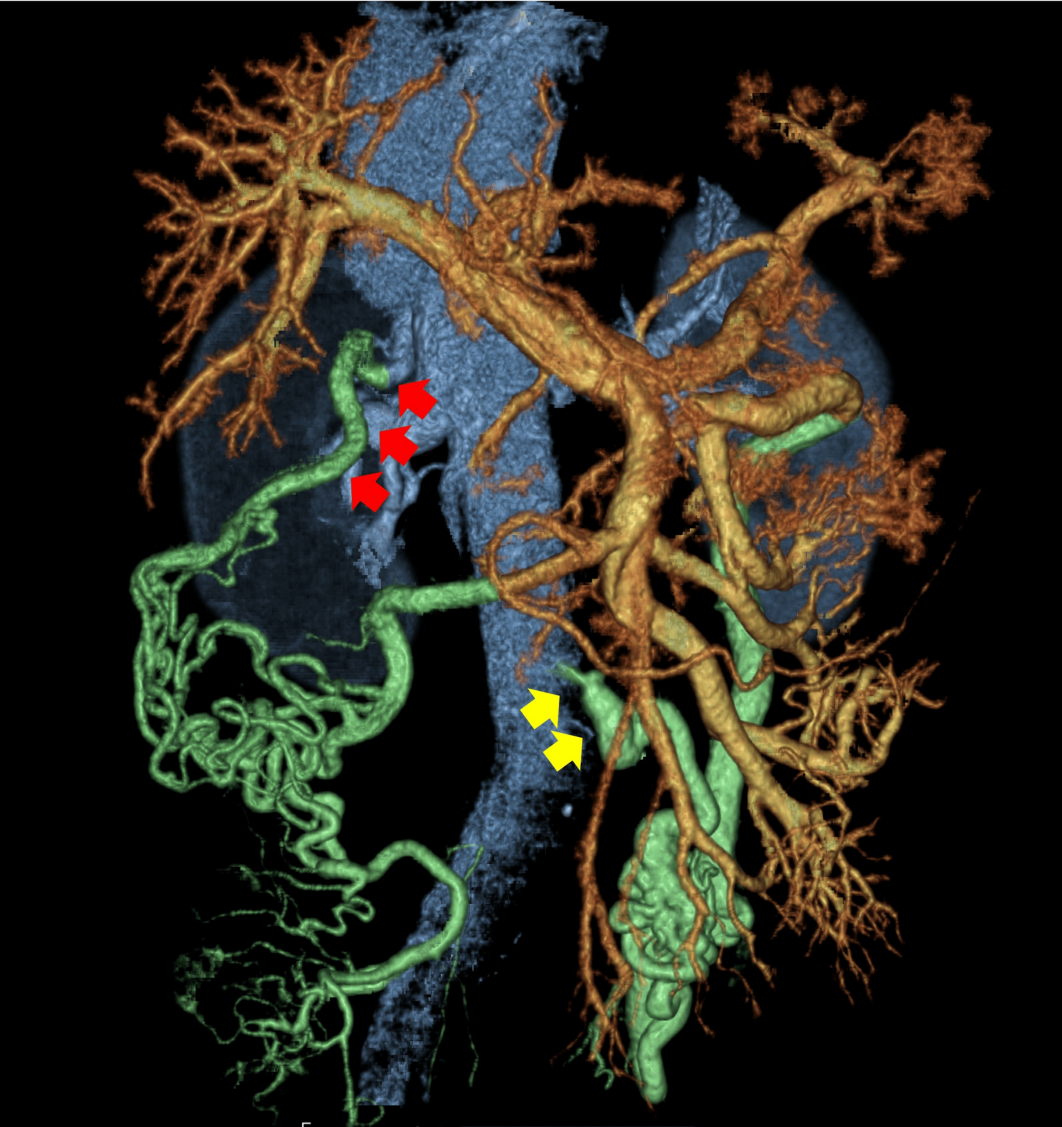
Contrast-enhanced CT Contrast CT showing abnormal blood vessels draining from the portal system into the inferior vena cava (yellow arrows) and the right renal vein (red arrows), indicating the formation of a portosystemic shunt.
